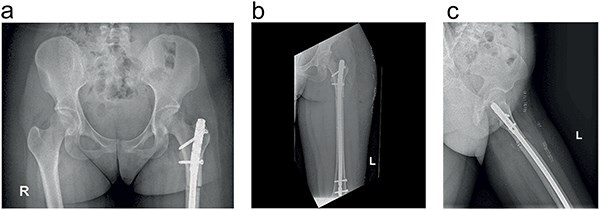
The postoperative AP (a and b) and lateral (c) radiographs after the subtrochanteric femoral osteotomy (derotation of 20°) and fixation achieved with an intramedullary nail locked proximal and distal are shown.
